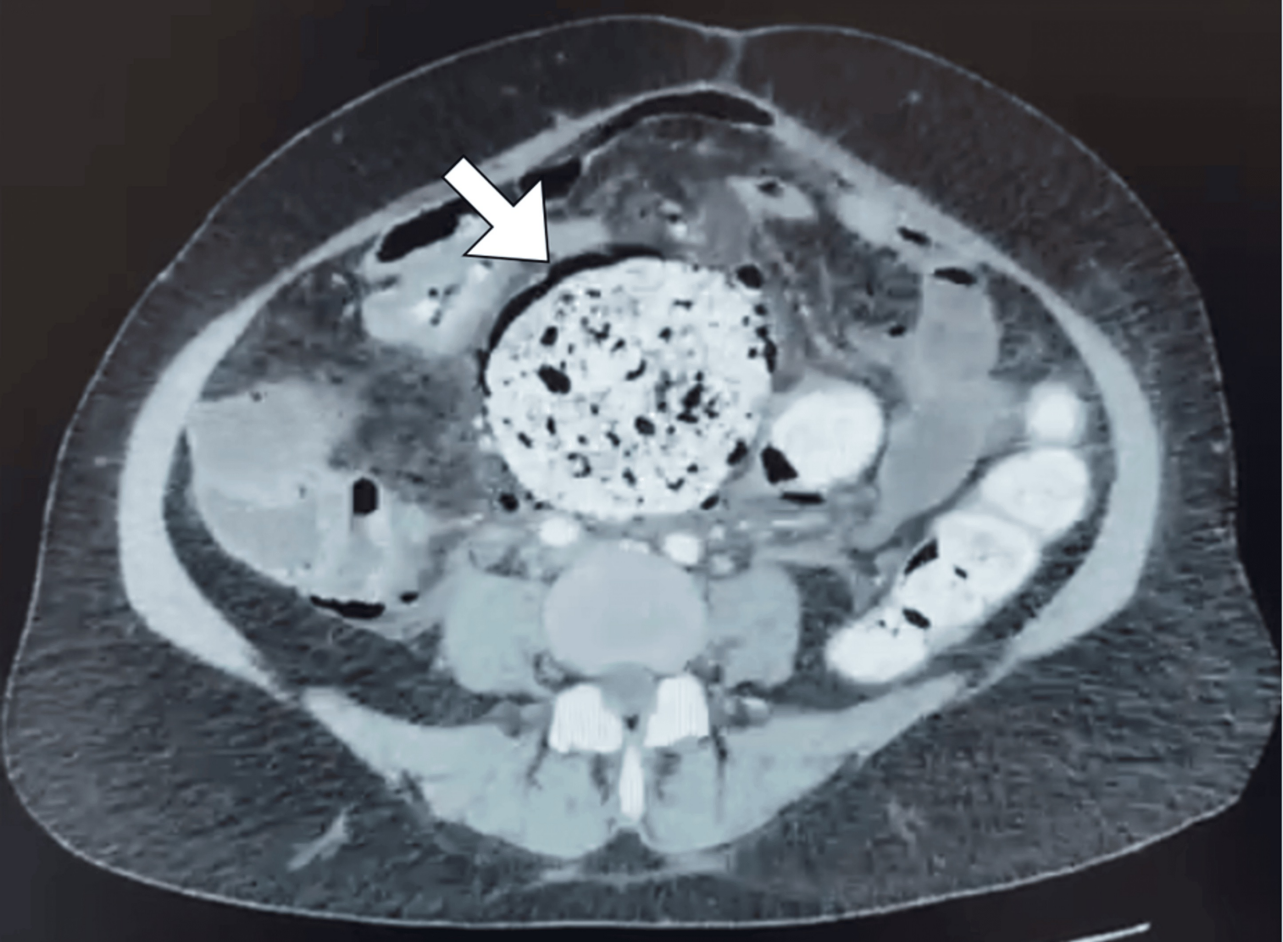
Axial image of contrast-enhanced CT scan of the abdomen The scan demonstrates a distended sigmoid colon (arrow) that is filled with fecal matter and surrounding multiple small air pockets suggestive of perforation. CT: computed tomography
